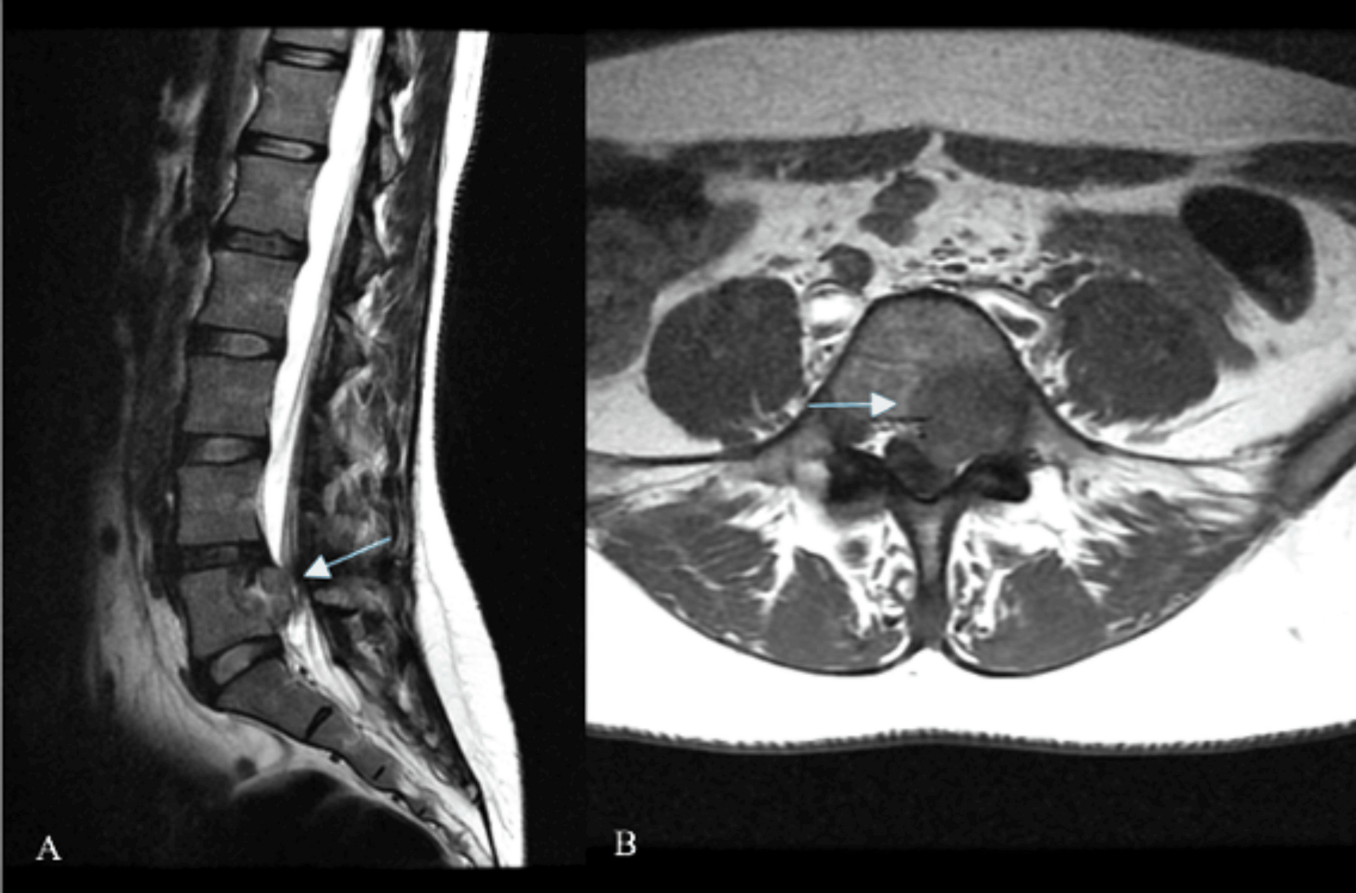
Lumbosacral MRI A: sagittal; B: axial views. The blue arrows indicate a lesion in the L5 vertebral body extending into the anterior and left margin of the central spinal.
